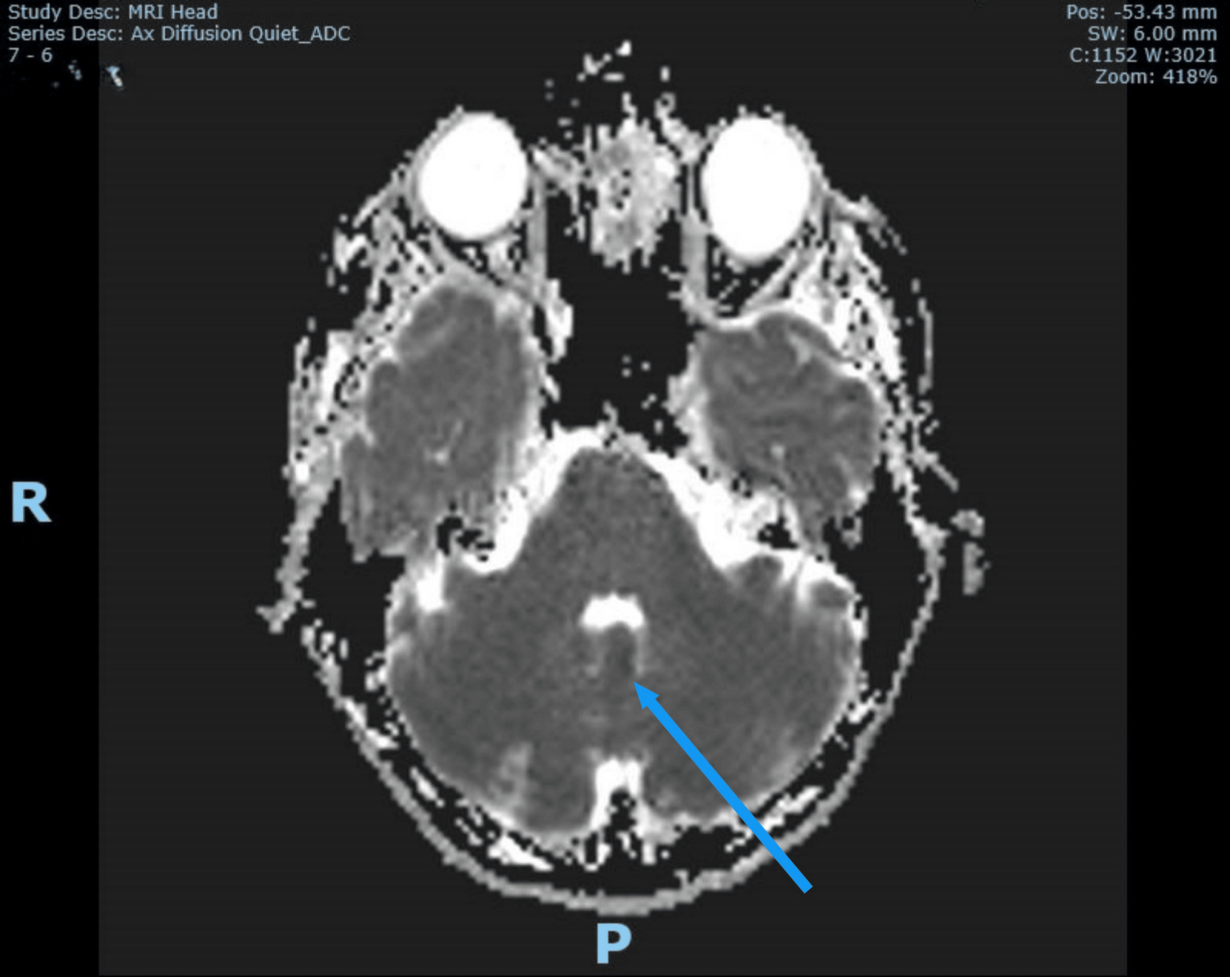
Corresponding MRI brain ADC confirming acute left cerebellar vermix infarct (blue arrow) MRI: magnetic resonance imaging ADC: apparent diffusion coefficient
